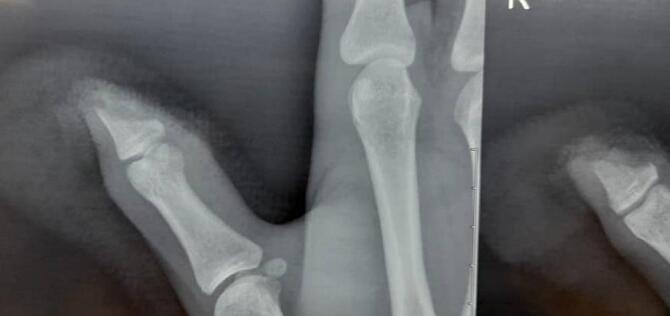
A degree of bone resorption can occur despite graft survival followed by a plain radiography (A-P).
